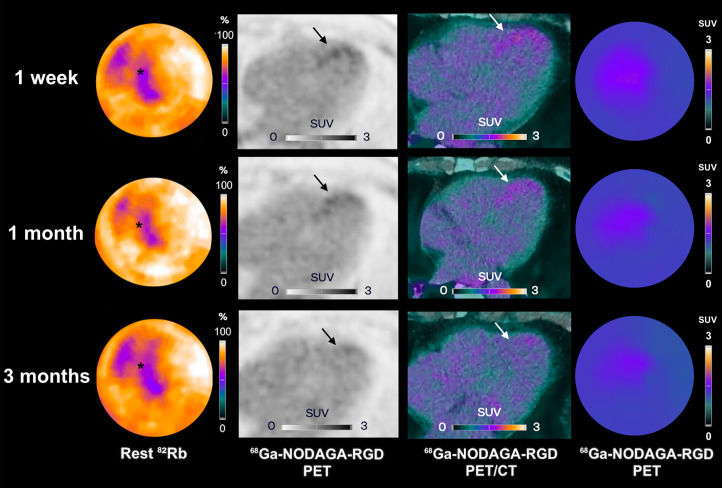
Participant example with decrease in α_v_β_3_ integrin expression levels (arrows) in infarcted myocardium (asterisks) after 1 wk to follow-up at 3 mo. Shown are rest ^82^Rb, ^68^Ga-NODAGA-RGD PET, and ^68^Ga-NODAGA-RGD PET/CT images at 1 wk (first row), 1 mo (second row), and 3 mo (third row) after STEMI.
